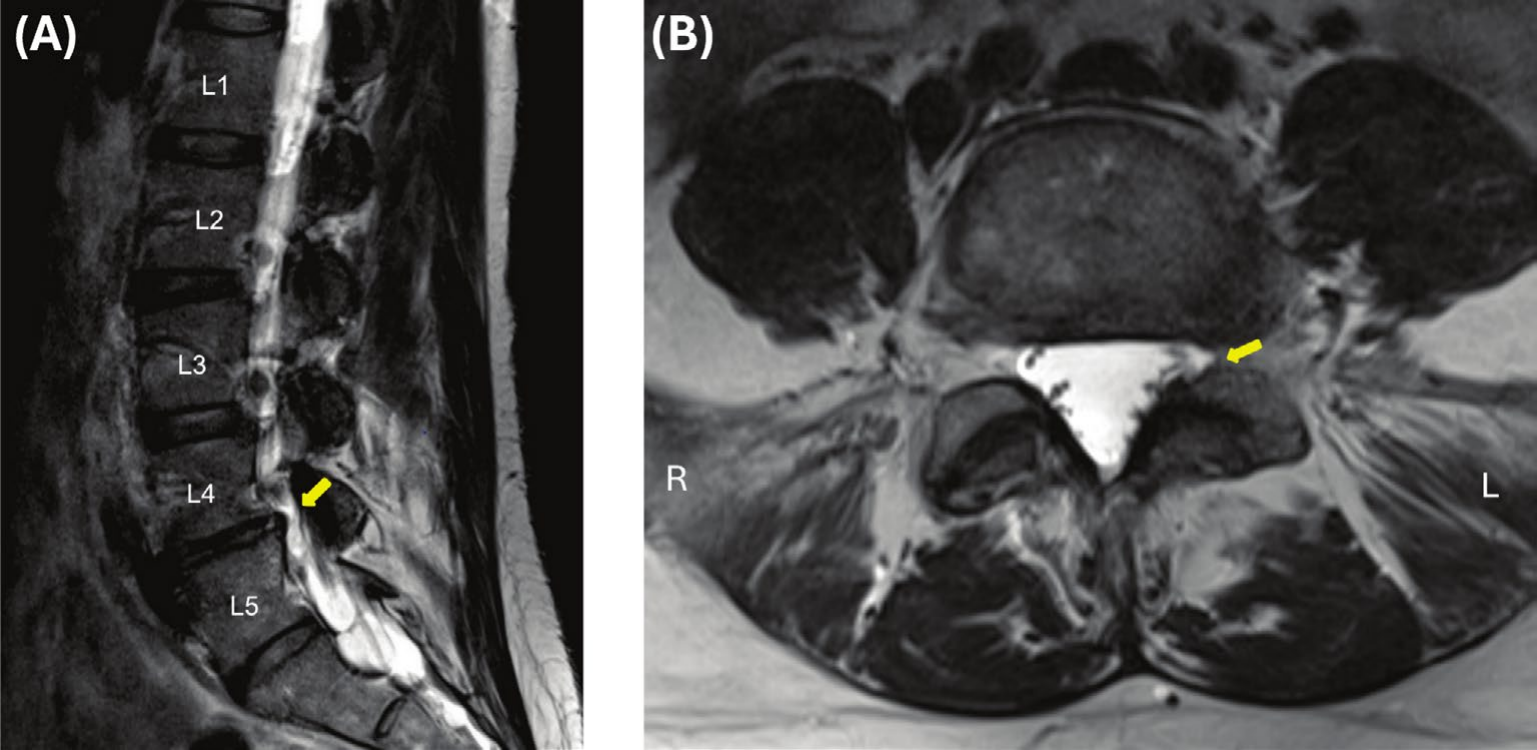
MRI of the lumbar spine without contrast, highlighting disc degeneration and disc height loss at L4–L5, accompanied by a small left foraminal disc protrusion that resulted in mild narrowing of the left neural foramen (Yellow Arrows) in (A) sagittal and (B) axial planes.
